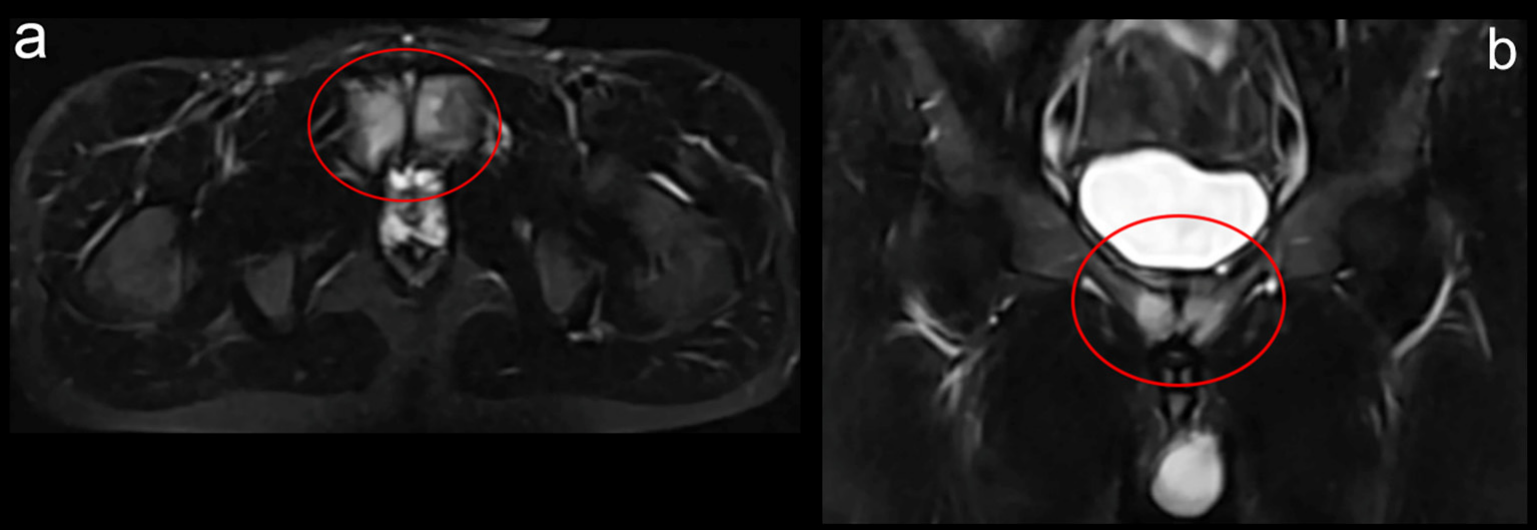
MRI investigation on the axial (a) and coronal plane (b). The exam highlights an increase of the phlogistic phenomenon of the pubic symphysis compared to Figure 4.
